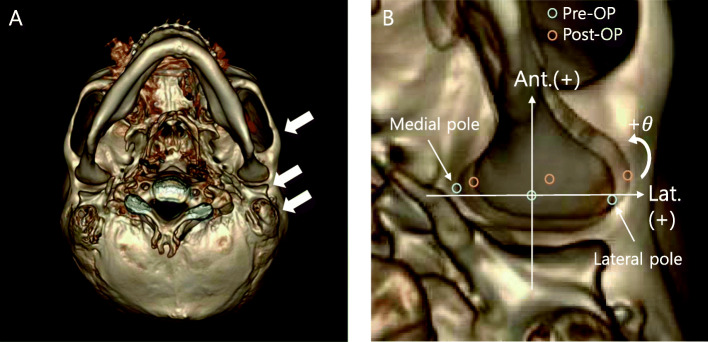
Axial view in 3D-CT: The reference points. A The anterior margin of the bilateral zygomatic arch, the posterior glenoid fossa, and the mastoid processes of both temporal bones. B Superimposed plane. the midpoint was designated on the axis connecting the medial and lateral poles of the condylar head. In the mediolateral displacement, positive values indicate the lateral direction. In the anteroposterior displacement, the positive values mean the anterior direction. For condyle rotation, the positive values mean inward rotation of condyle head
